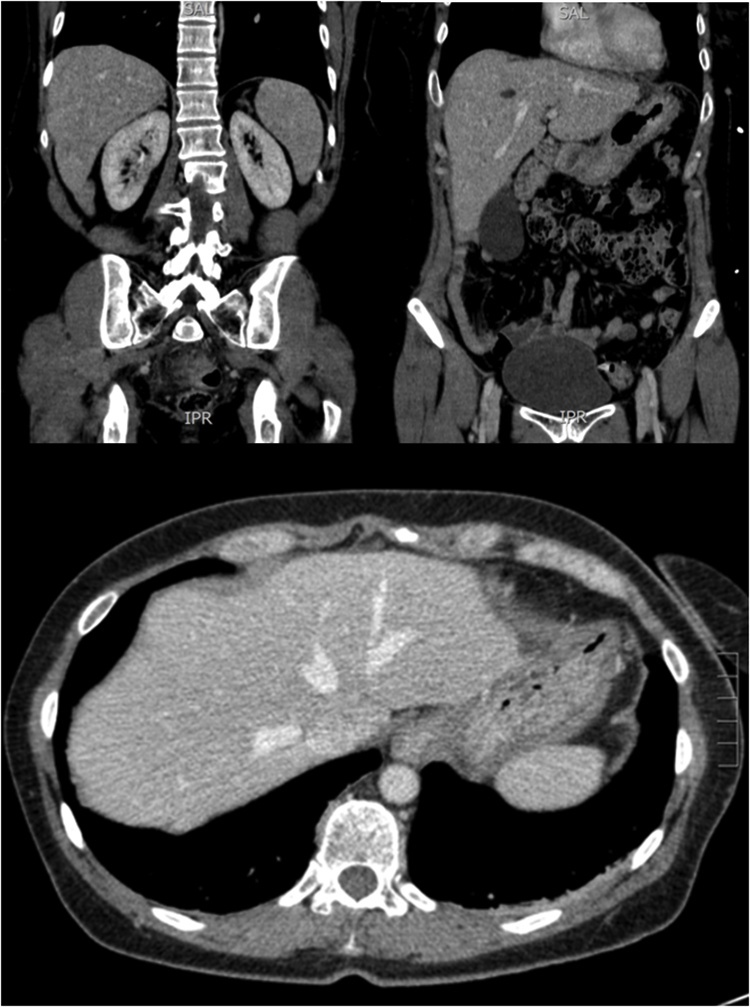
CT images of the same patient 3 years prior to presentation.
